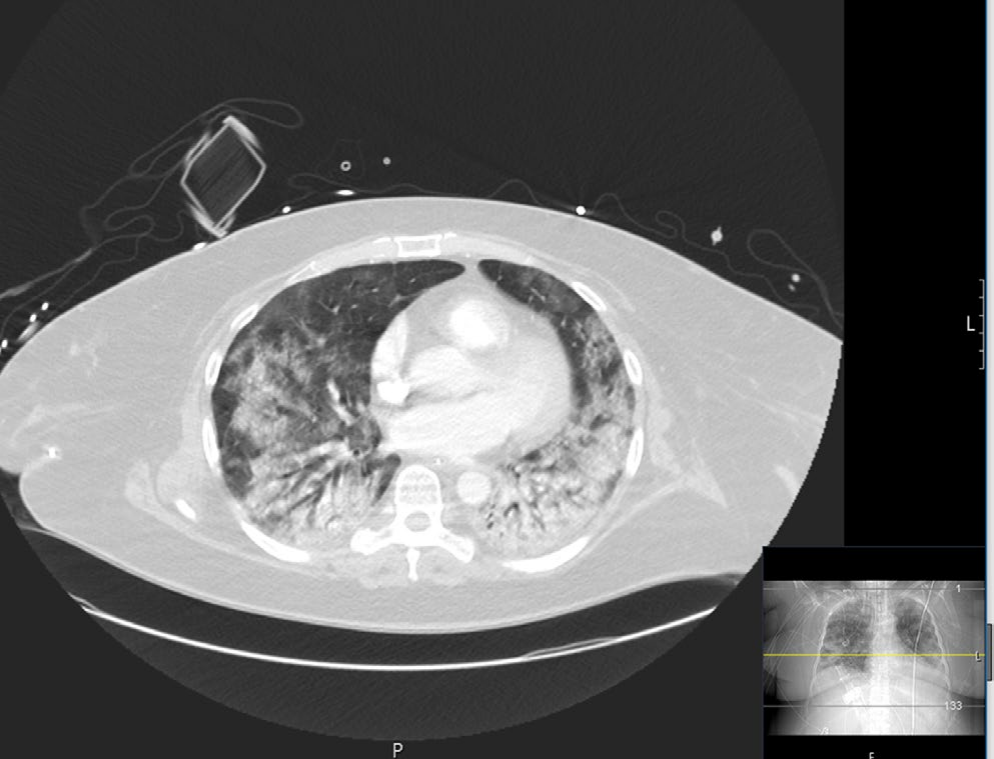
CT lung scan on day 20: CT scan shows severe bilateral infiltrates with lower and dorsal segments heavier affected
